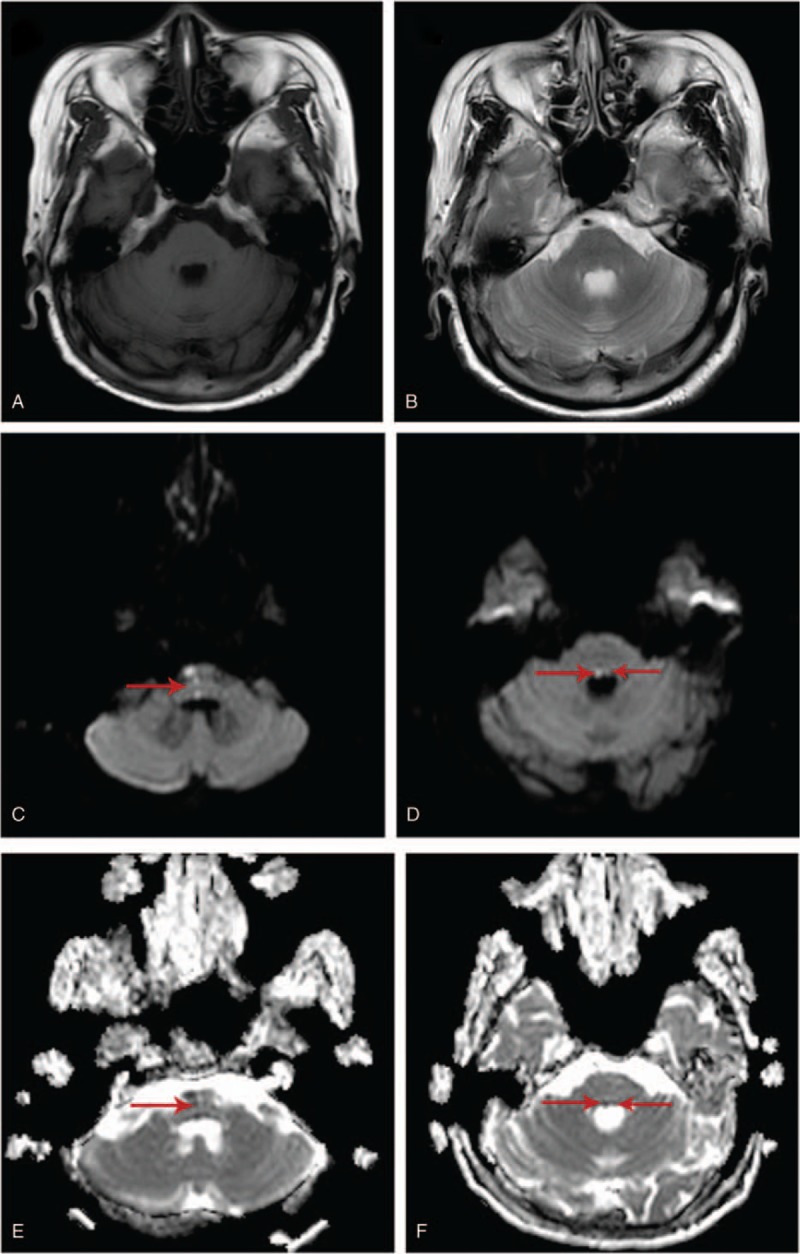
Axial T1-weighted (A) and T2-weighted (B) images was consistent with no definite abnormality. Axial diffusion-weighted image (C and D) demonstrated the restricted diffusion in the territory of bi-lateral pontine tegmentum and another bigger lesion in the right of the pons near the midline, whose apparent diffusion coefficient (ADC) was low, meanwhile. ADC = apparent diffusion coefficient.
